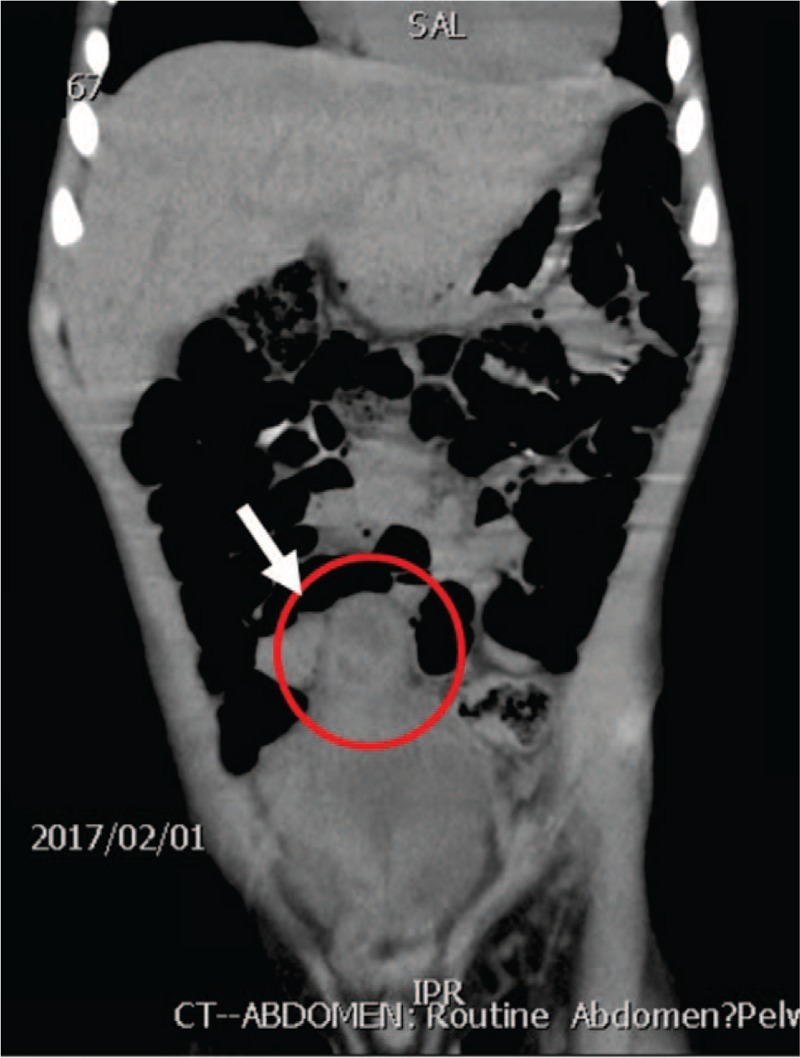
Sagittal and coronal views of abdominal CT (non-contrast): the cystic-like mass lesions located at the apex of the bladder; heterogeneously enhanced cyst inclusion (arrow). CT = computed tomography.
